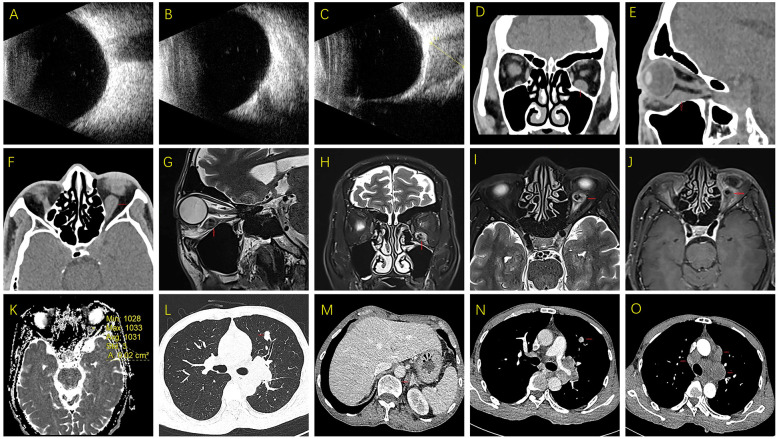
Ophthalmic B-scan ultrasonography, plain orbital CT scan, orbital magnetic resonance imaging, plain chest CT scans, abdominal CT scans, and contrast-enhanced chest CT scan. The images show vitreous opacities in both right (A) and left eye (B). A lesion in the left orbit involving the inferior rectus muscle was revealed. The yellow double-headed arrow indicates the measured width of the inferior rectus muscle belly (8.40 mm) (C). (D–F) Axial CT images in the soft tissue window demonstrate diffuse thickening of the left inferior rectus muscle, most prominently involving its muscular belly (red arrows). (G–I) Axial T2-weighted MRI reveals a well-circumscribed annular hyperintense lesion located within the medial aspect of the left inferior rectus muscle, measuring approximately 1.1 × 0.8 cm, with patchy intralesional hypointensities. The adjacent tendon exhibits swelling and T2 hyperintensity suggestive of edema, while the surrounding orbital fat is preserved without abnormal signals (red arrows). (J) Contrast-enhanced T1-weighted MRI shows peripheral ring-like enhancement with a central non-enhancing region (red arrow). (K) ADC map demonstrates restricted diffusion, with values ranging from 1.03-1.15 mm^2^/s. (L) A soft tissue density nodule in the anterior segment of the left upper lobe with clear boundaries. Lobulated margins, spiculations, and vessel convergence sign are identified at the edge of the nodule (red arrow). (M) The left adrenal gland appears thickened (red arrow). (N) A markedly enhancing nodule with heterogenous enhancement in the anterior segment of the left upper lobe (red arrow). (O) Multiple enlarged lymph nodes in the left hilum and mediastinum (red arrow).
